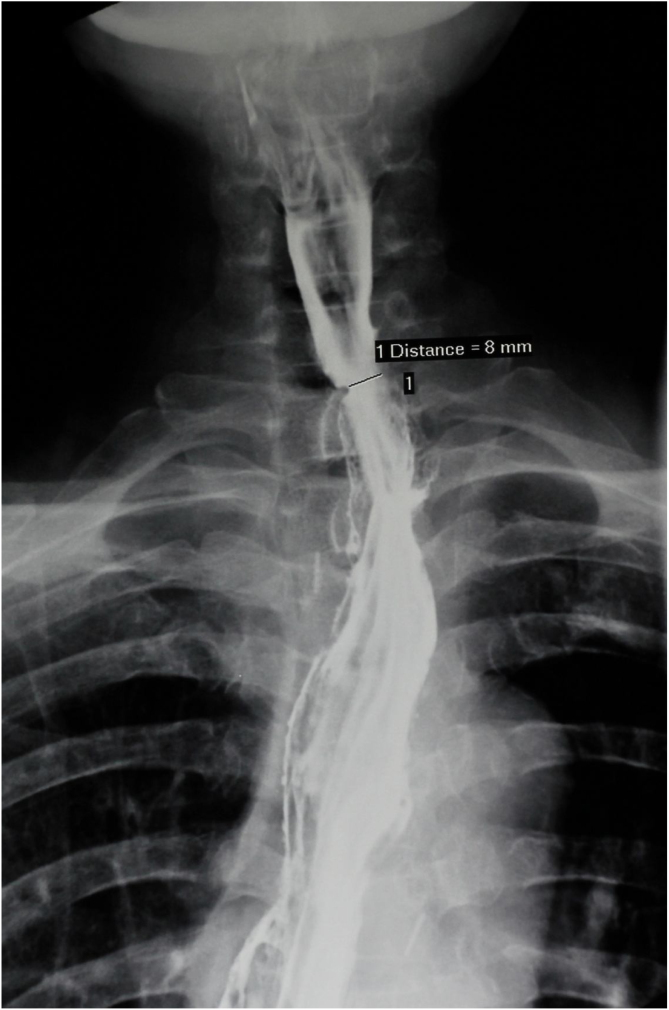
Barium swallowing X-ray 10 months later demonstrated a smooth alimentary tract without any anastomotic stricture.
